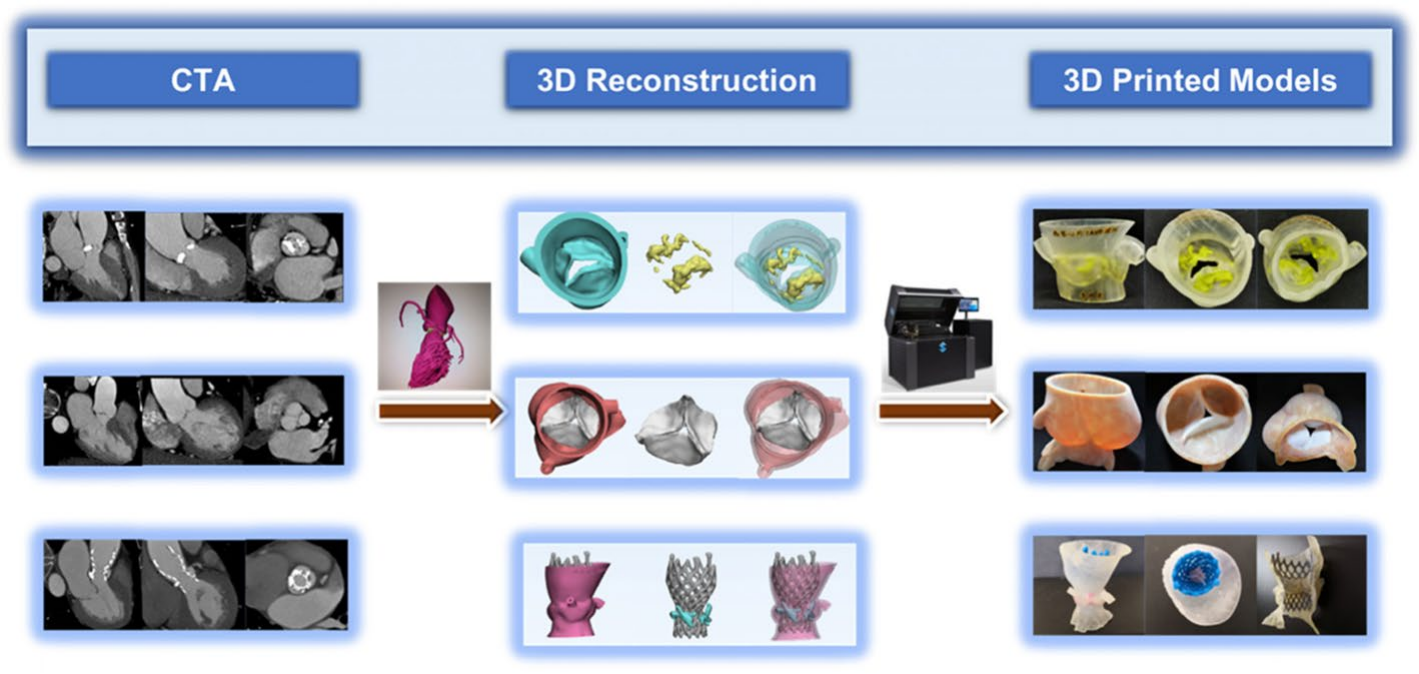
Pre- and postprocedural computed tomography angiography (CTA) data were used for the process of 3-dimensional (3D) printing
